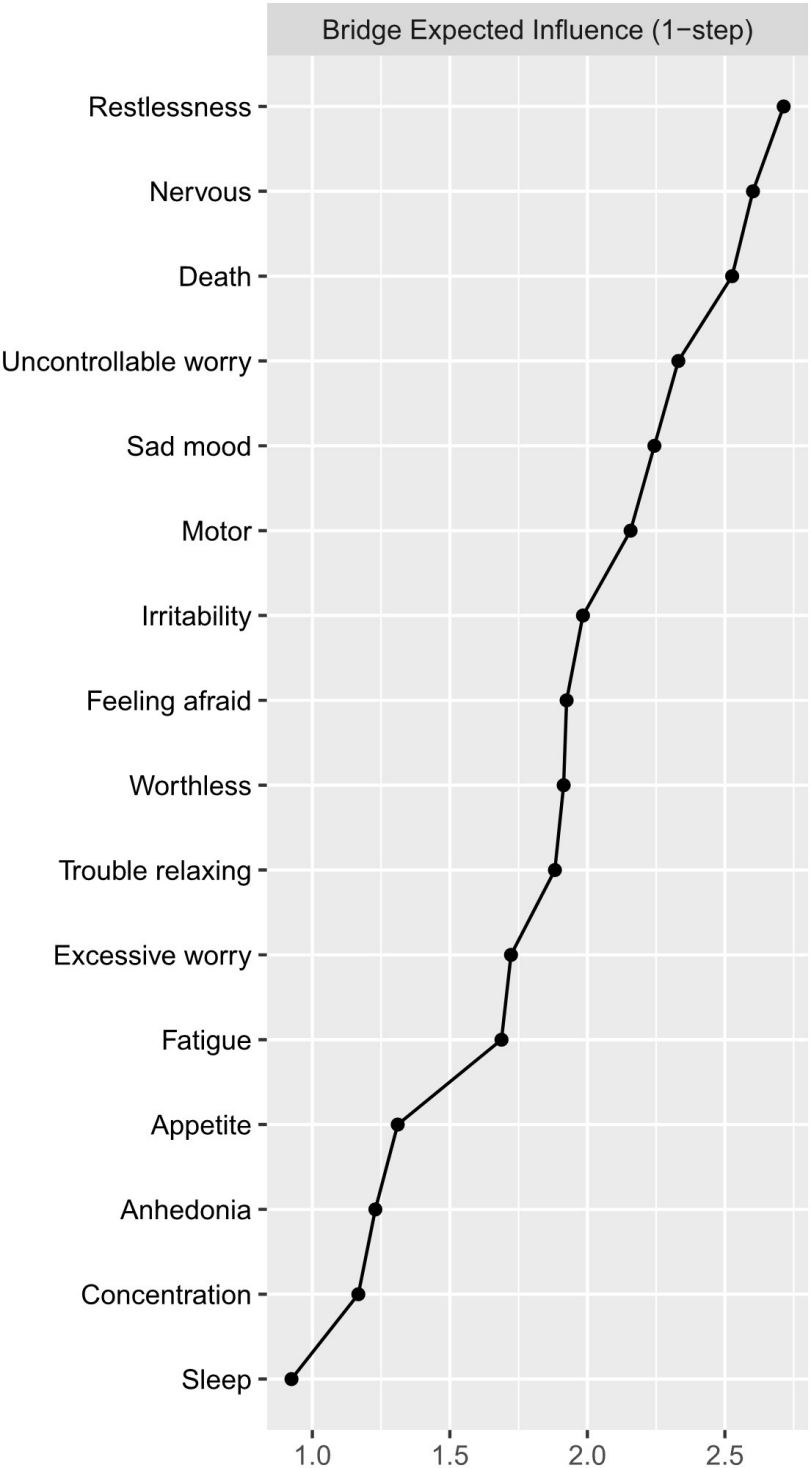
The bridge expected influence plot. The X-rays represented the bridge expected influence of each node. Nodes with higher bridge expected influence are recognized as bridge symptoms that drove the comorbid depression and anxiety symptoms.
